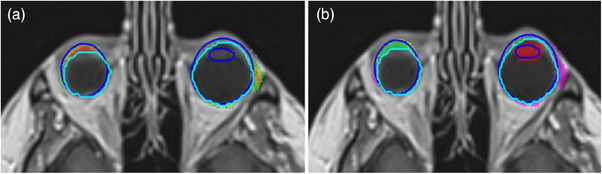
Axial T1w‐Gd MRI showing the failure of AutoConfidence prediction to detect the missing autosegmentation for the left lens, resulting in false‐negative. Blue represents the gold standard, while light blue represents EM‐HQ autosegmentation. (b) Four‐color‐map showing regions of TP (green), FP (pink), and GDC modified FP (yellow) relative to the differences‐to‐gold‐standard, for the ACo prediction.
